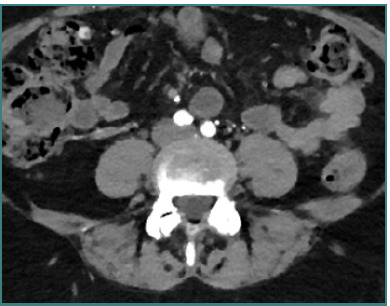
Axial view of L4-L5 level on CTA
